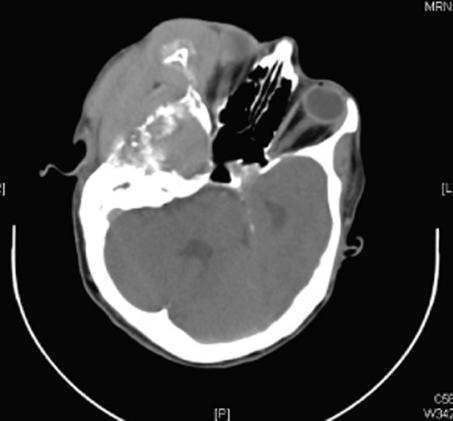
Axial CT scan image showing tumour invading the orbit, right side of skull base and destroying the bones. This is suggestive of aggressive tumour.
